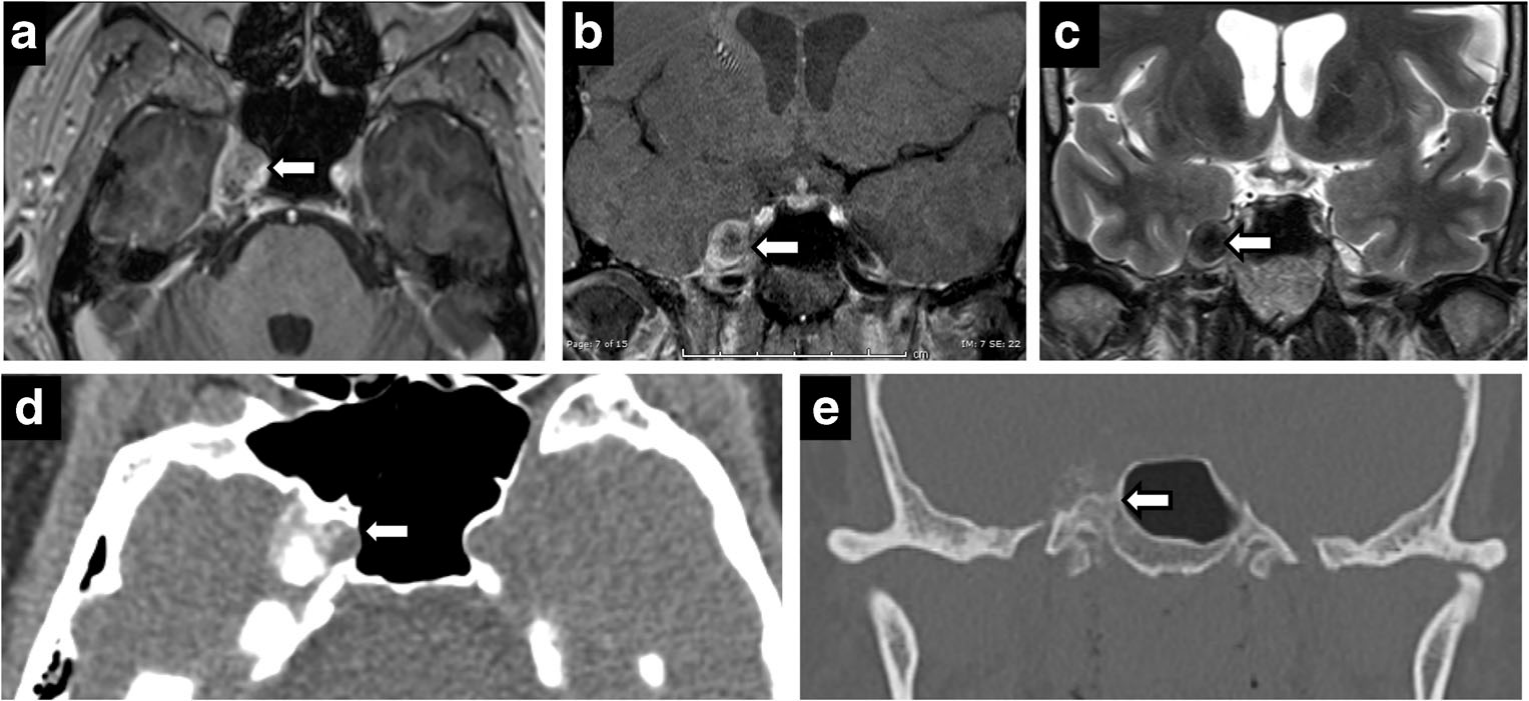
Meningioma (intrinsic). Axial C+ (a), coronal C+ (b), coronal T2 (c), axial CT (d), coronal CT (e). Circumscribed enhancing right Meckel’s cave mass—hypointense on T2. CT shows calcifications with hyperostosis of adjacent bone
